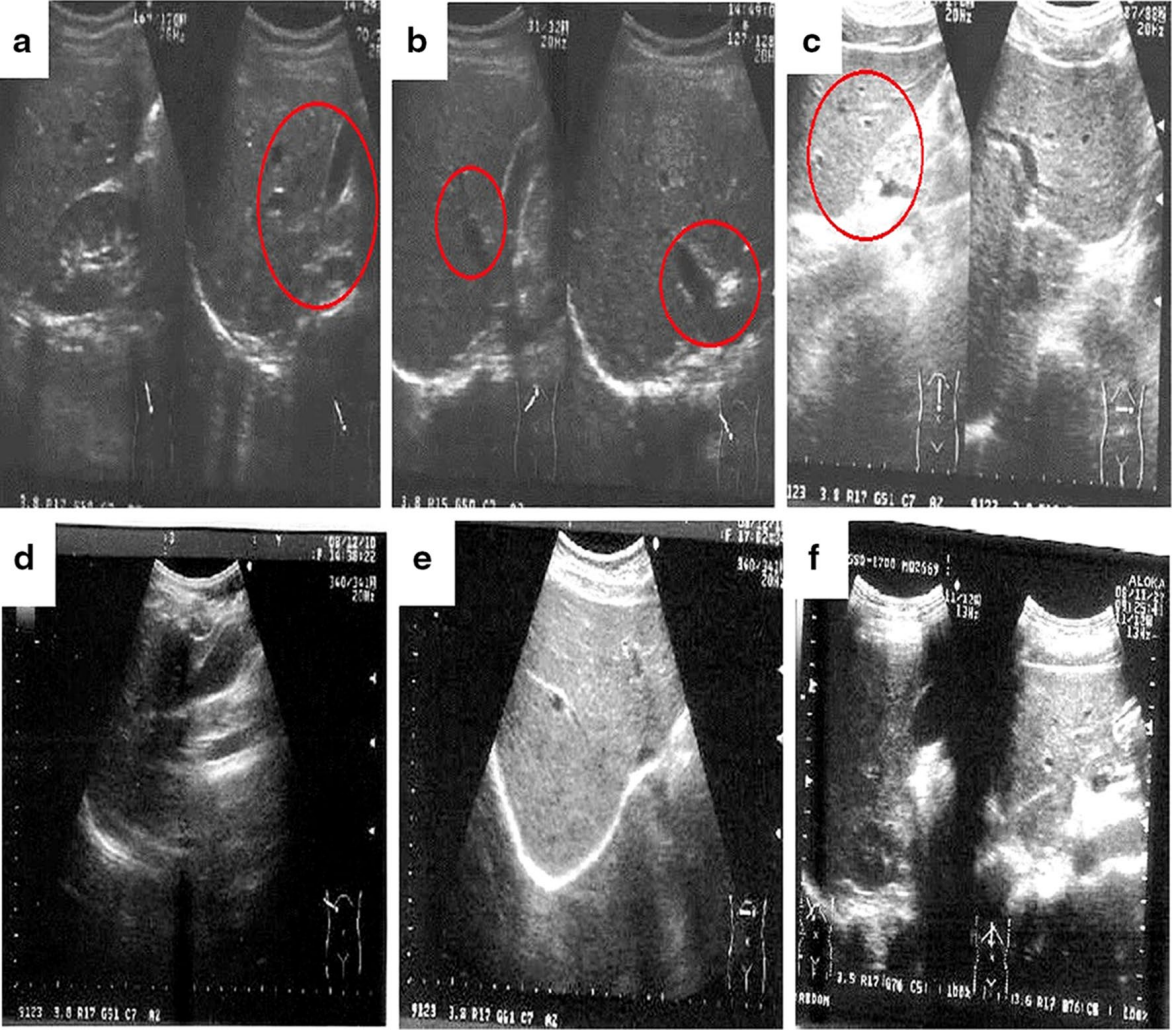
The Abdominal ultrasonic image of SWT treated healthy participants. a–c represented participant no. 3, 9 and 28 at baseline with mild fatty liver, gall bladder stone and splenomegaly respectively (indicated with *arrow mark*). d–f represents participant no. 3, 9 and 28 at 6th month of SWT treatment with normal inference (no signs of fatty liver, gall bladder stone and splenomegaly)
